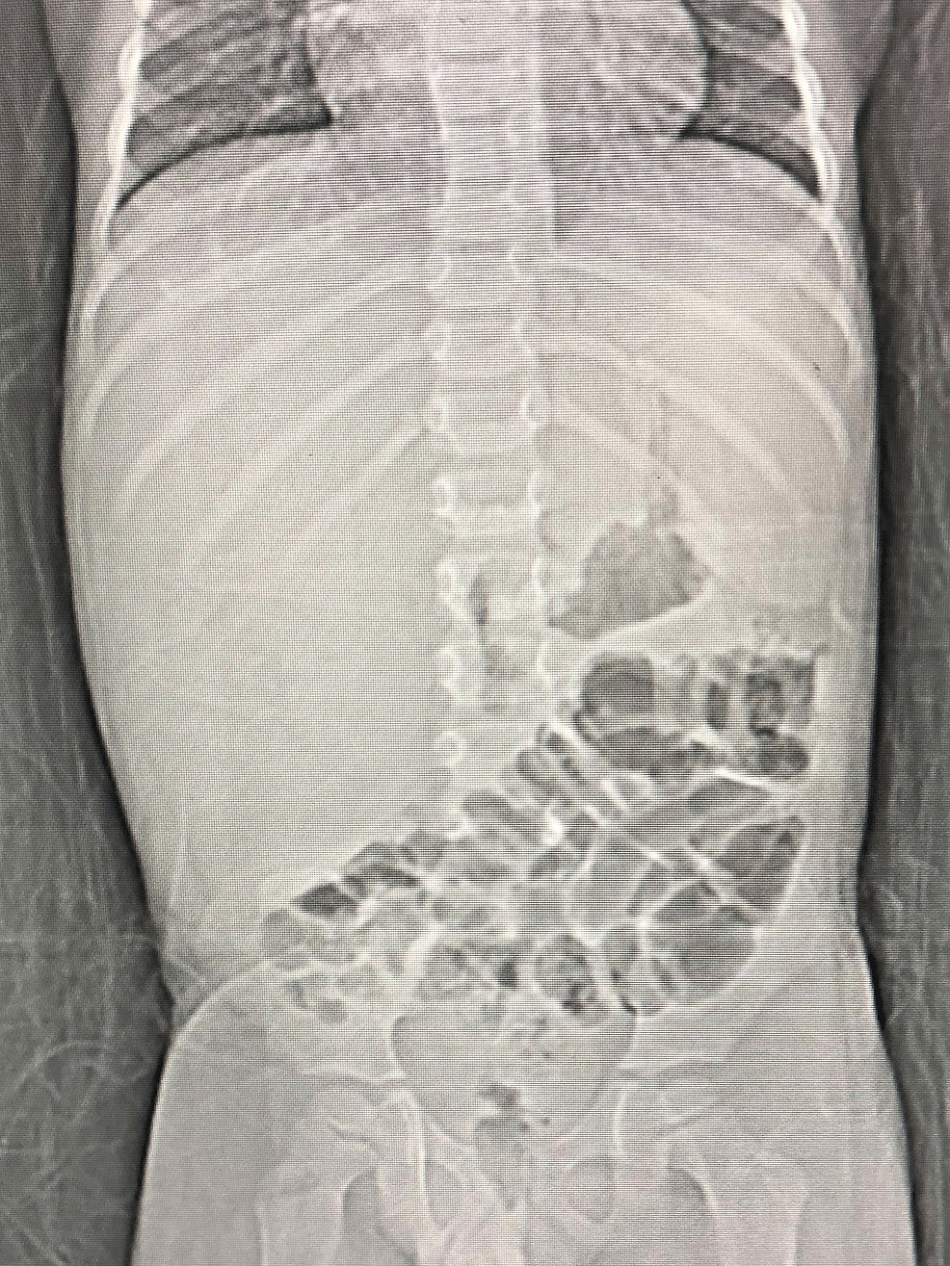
Abdominal and pelvic CT with contrast.
